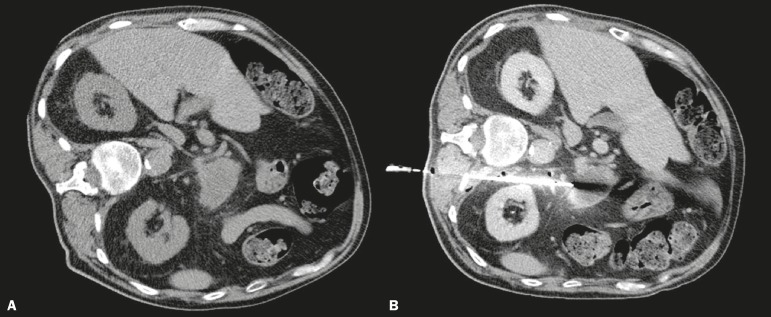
CT-guided percutaneous biopsy using direct access. A:
